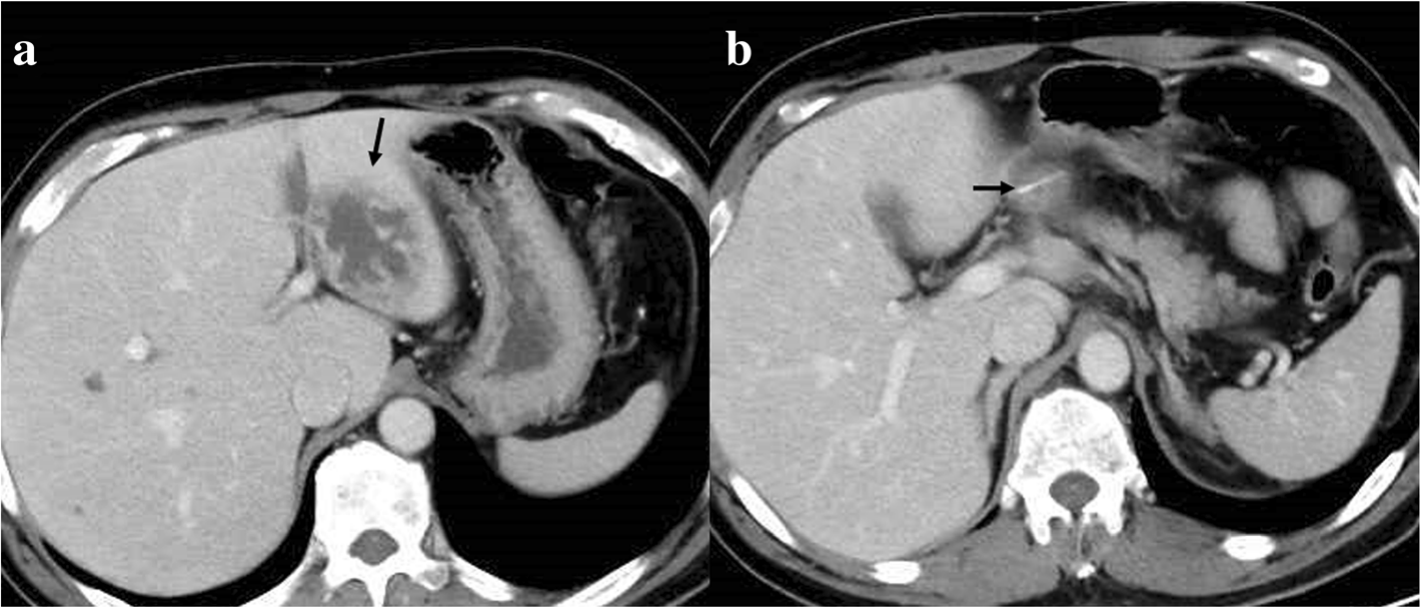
Abdominal contrast-enhanced computed tomography findings. a A lesion with coexisting low- and high-density areas (black arrow) was found in the left lobe of the liver. b A hyperdense linear body (black arrow) was found in the liver abscess.
